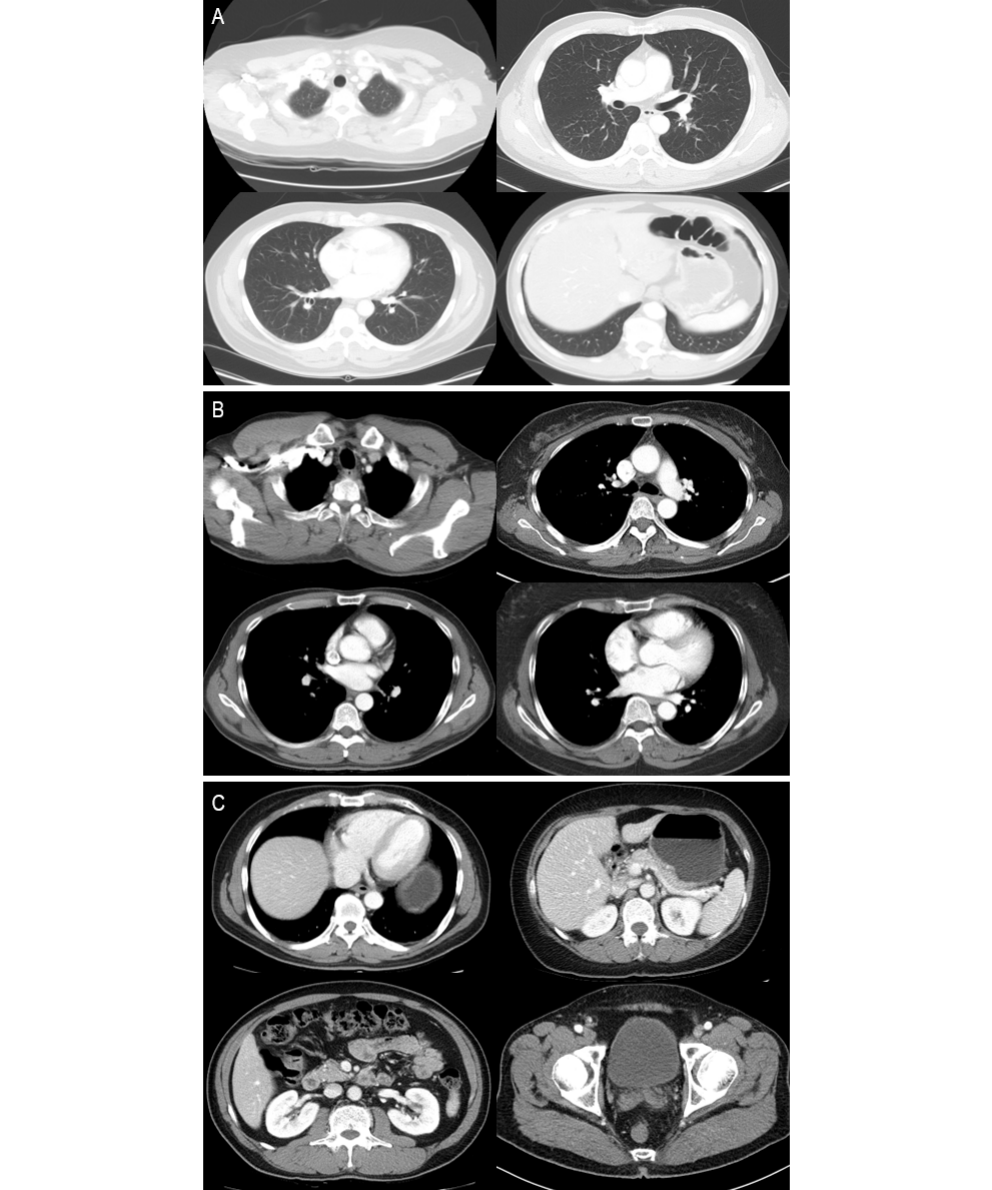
Synthetic high-resolution body computed tomography images. A. Chest computed tomography images-lung window. B. Chest computed tomography images-mediastinal window. C. Abdominopelvic computed tomography images.
